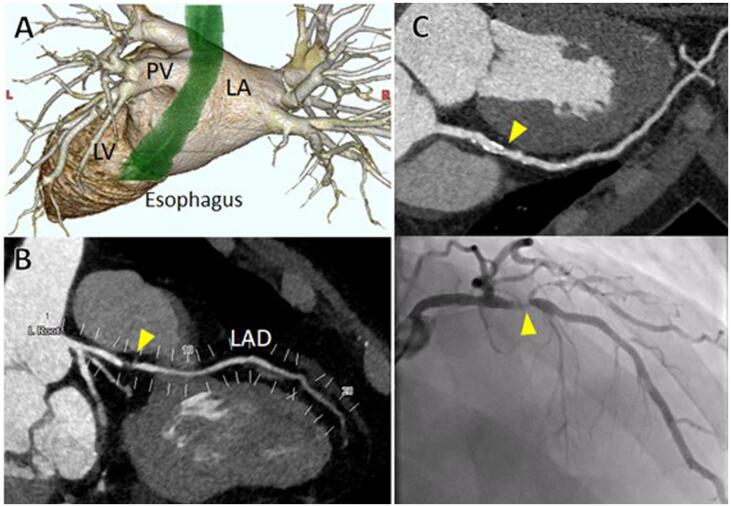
Representative computed tomography (CT) and coronary angiography images A. Three-dimensional construction of the left atrium, pulmonary veins, and esophagus (green). LA, left atrium; PV, pulmonary vein; LV, left ventricle. B. A representative case of stenosis in the LAD assessed by axial and cross-sectional images of contrast-enhanced CT in a patient with a CHADS_2_ score of 3 points. Note the severe coronary stenosis in the proximal LAD (yellow arrowhead). LAD, left anterior descending artery. C. A representative case of a calcified LAD that was inconclusive for coronary artery disease on contrast-enhanced CT in a patient with a CHADS_2_ score of 1 point (yellow arrowhead in the upper panel). Coronary angiography reveals stenosis in the LAD (yellow arrowhead in the lower panel). LAD, left anterior descending artery. (For interpretation of the references to colour in this figure legend, the reader is referred to the web version of this article.)
